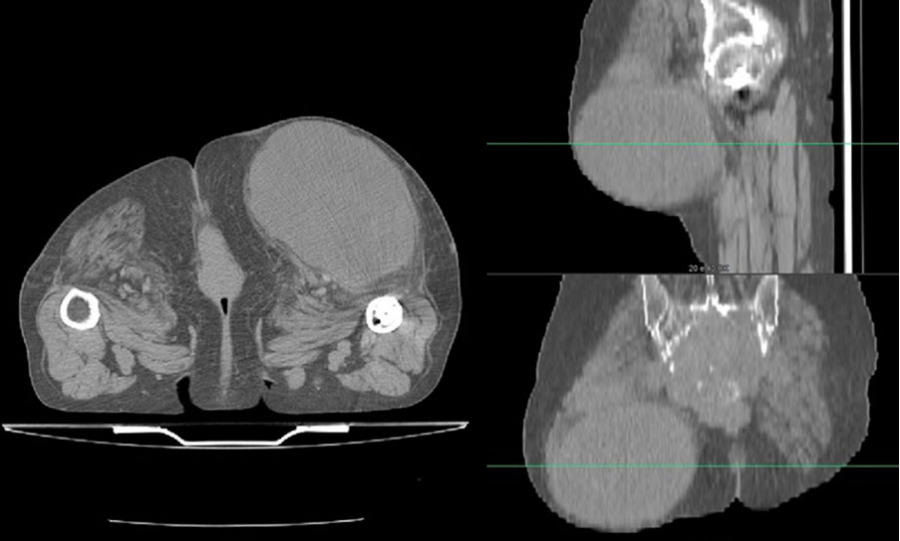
Axial, sagittal, and coronal CT scans of a huge chordoma, showing a large swelling infiltrating the right gluteal region and the ipsilateral thigh root.
